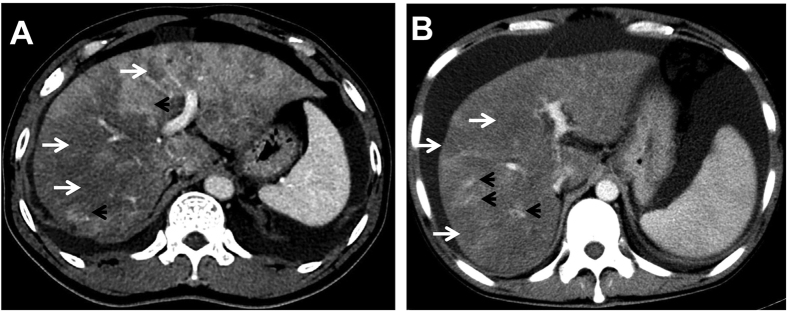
Contrast-enhanced CT scans showed that heterogeneous hypoattenuation and patchy liver enhancement were two most important radiological signs of PAs-induced HSOS. Heterogeneous hypoattenuation represented heterogeneous hypoattenuated, or low-density areas appear darker (arrow); patchy liver enhancement was inhomogeneous enhancement of liver parenchyma (arrowhead).
